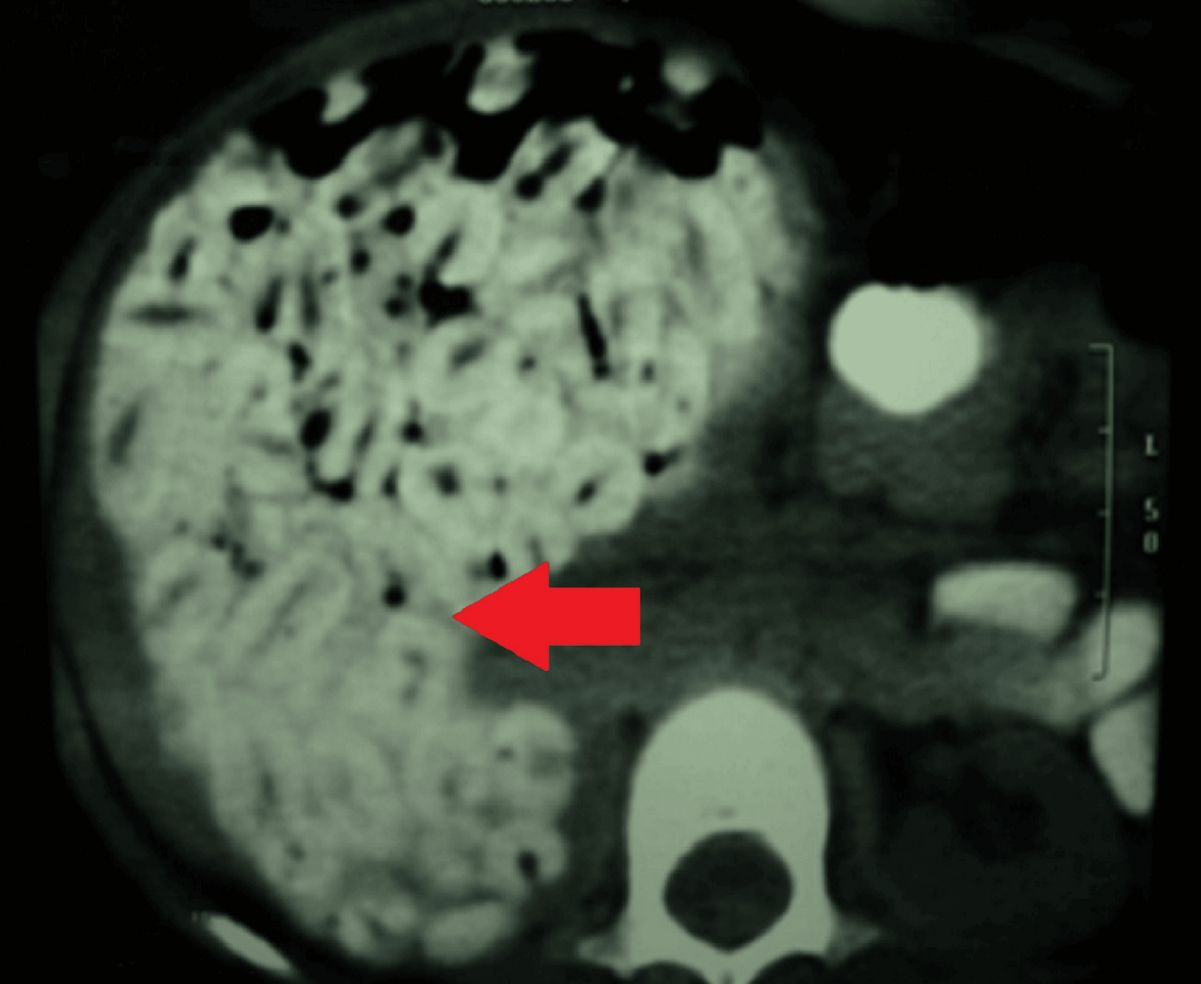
CT-scan abdomen with contrast CT scan with axial view of the dilated duodenum filled with dates’ seeds. Note the distinctive shape of the seed with a central, longitudinal groove (red arrow).
